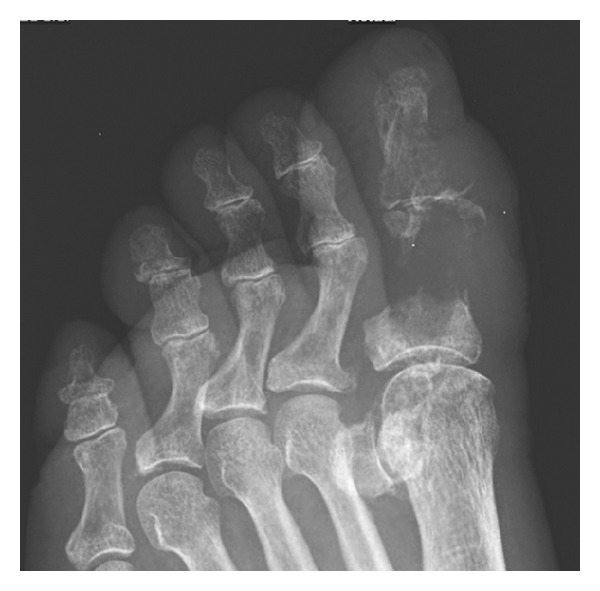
X-ray of right foot showing erosive and lytic lesion with cortical disruption in the proximal phalanx of the right hallux associated with soft tissue swelling.
